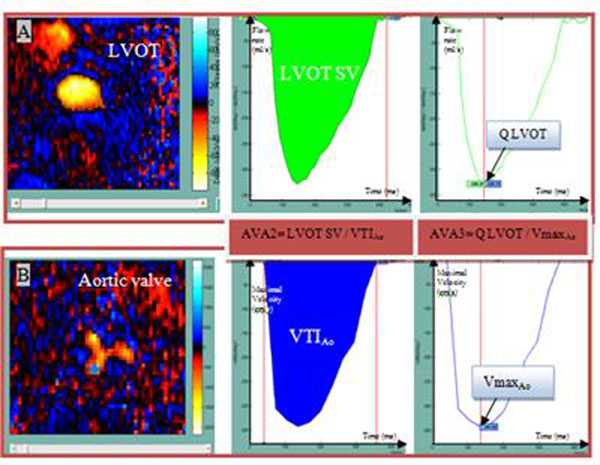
A) Example of left ventricular outflow tract (LVOT) segmentation on a velocity PC MR image, flow rate curve, whose integral corresponds to LVOT stroke volume (LVOT SV) and whose peak corresponds to Q LVOT Q; B) Example of aortic flow segmentation on a velocity PC MR image, transaortic maximal velocity curve, whose integral corresponds to VTI_AO_ and whose peak corresponds to Vmax_AO_. AVA2 is the ratio between the two integrals. AVA3 is the ratio between the two peaks.
